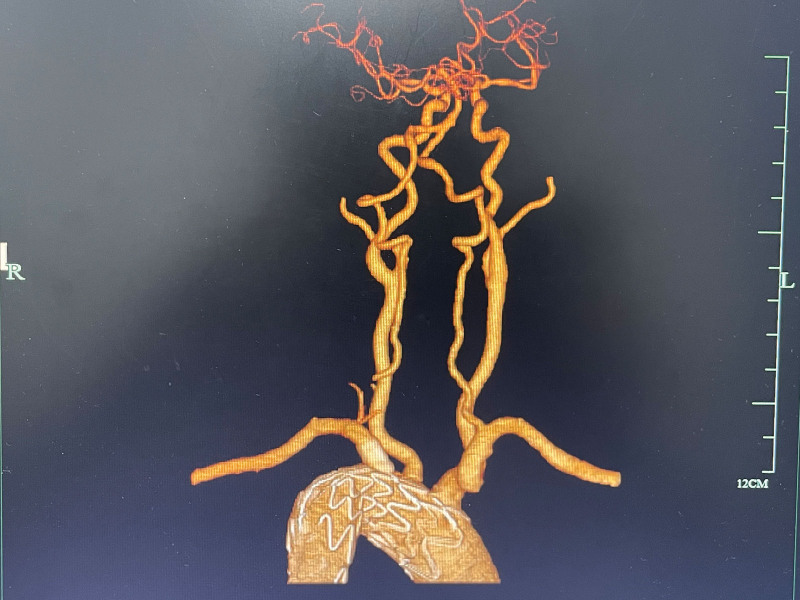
CTA indicated good postoperative outcome at 3 mo follow-up after hybridization. CTA = computed tomography angiogram.
